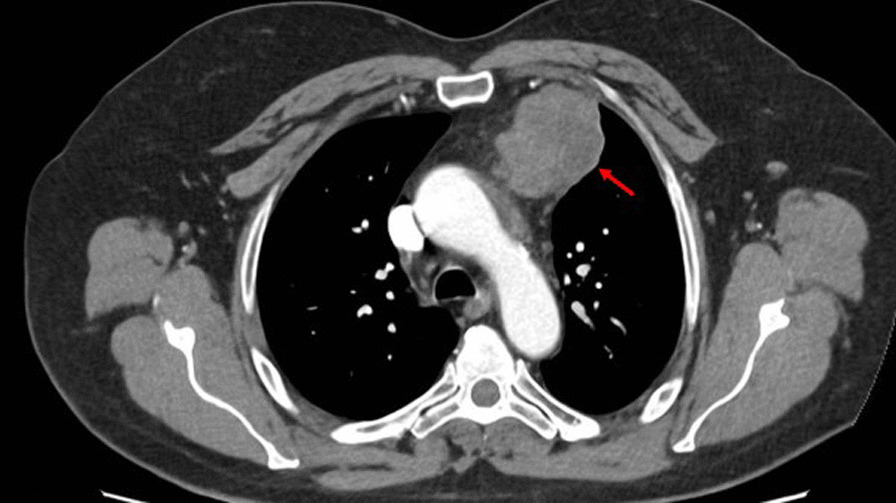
Axial slice of contrast-enhanced CT slice of the thorax demonstrating the mediastinal mass (red arrow)
